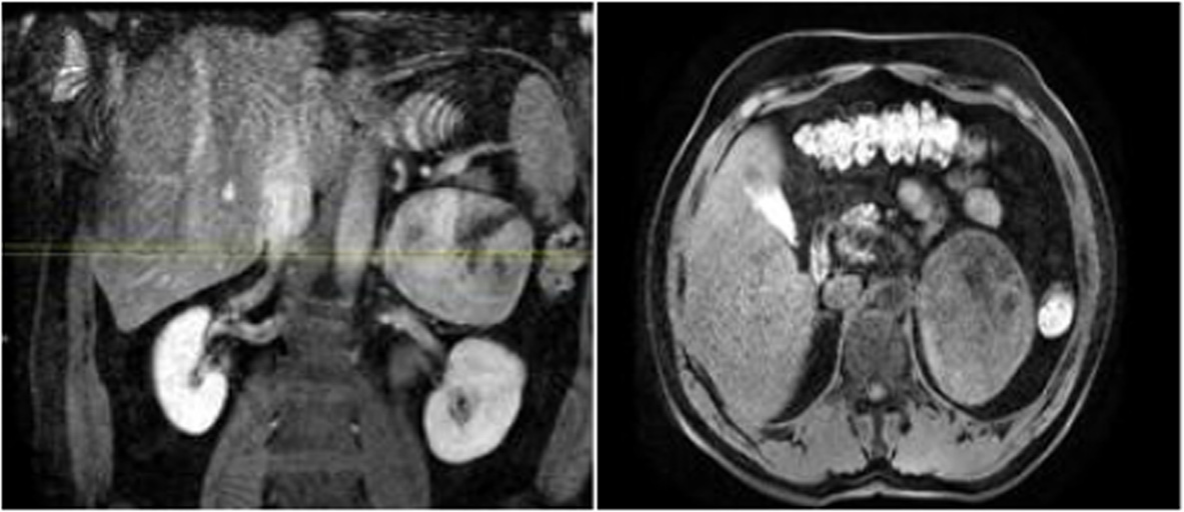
Dynamic magnetic resonance imaging scans (left adrenal mass).
